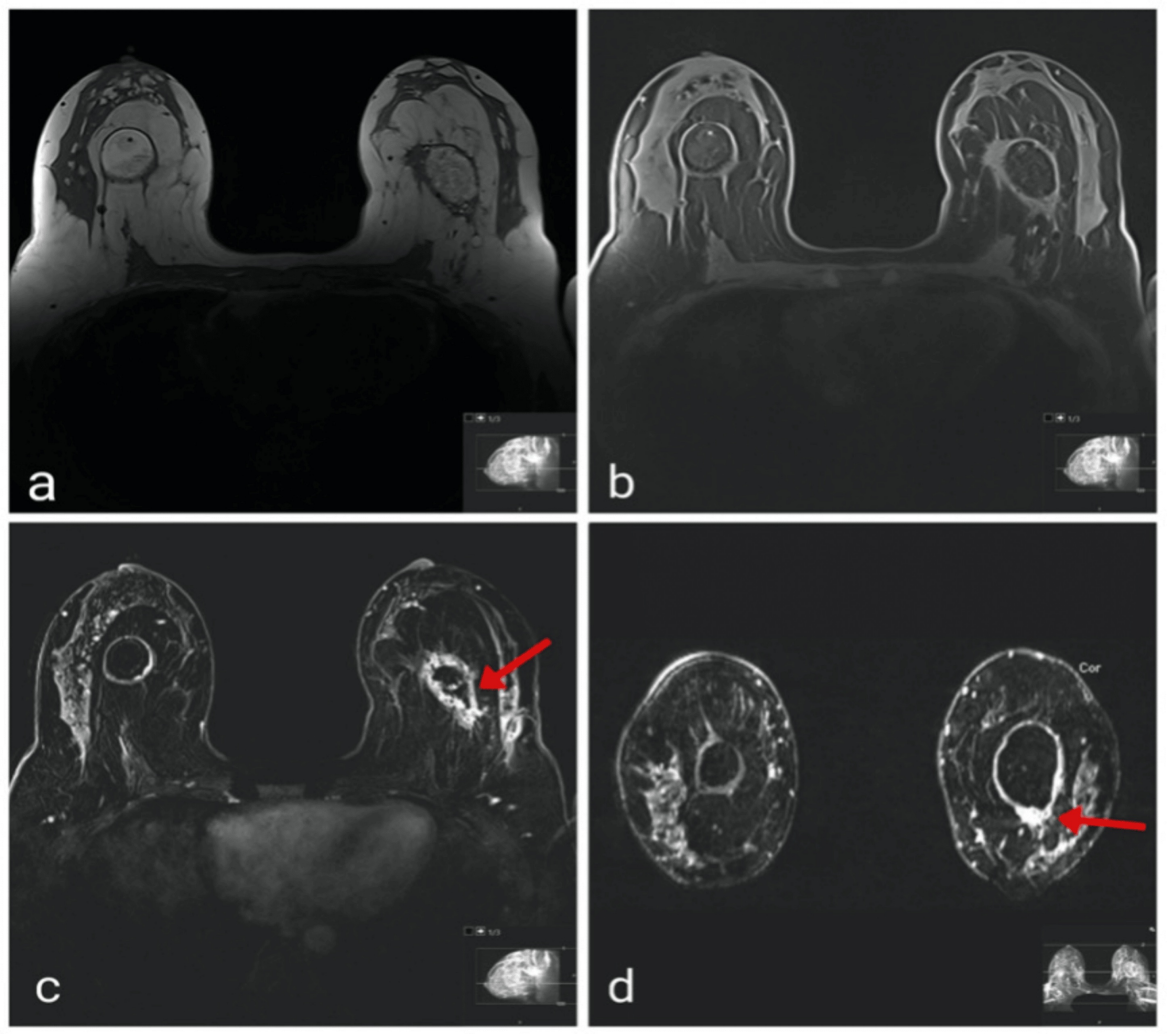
Pre-lumpectomy MRI T1 non-fat-saturated (a), T1 fat-saturated (b), axial (c), and coronal (d) subtraction MRI sequences showing bilateral oval, fat-containing masses. The left-sided lesion demonstrates a thick, enhancing wall. The red arrow indicates a suspicious, irregular, enhancing mass at the inferior margin of the fat necrosis in the left breast.
